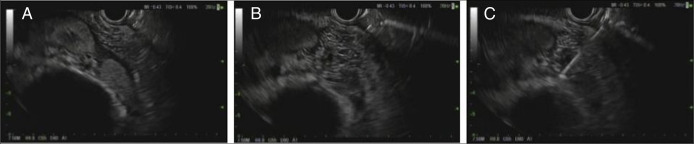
(A) Endoscopic ultrasound demonstrating right renal parenchyma. (B) Irregular, right renal mass. (C) Renal mass fine needle biopsy.
